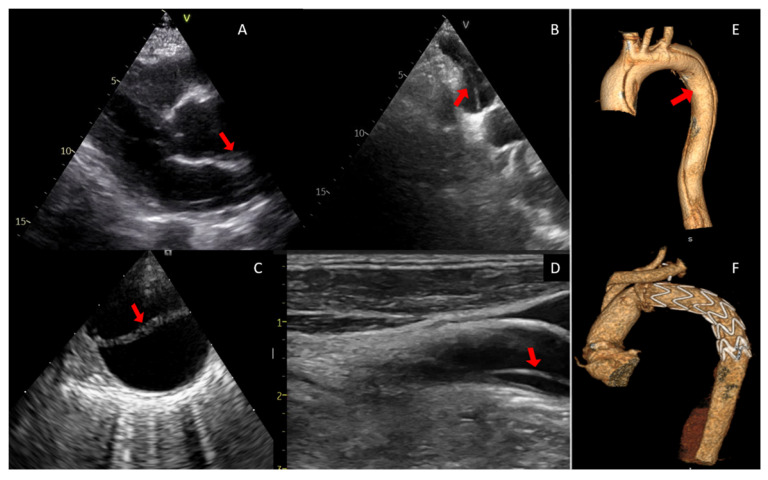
(A) TTE, parasternal long-axis view showing the presence of an intimal flap in the ascending aorta (arrow) and the correct anatomical conformation of the aortic root. (B) Intraoperative suprasternal section of the aortic arch showing an intimal dissection flap and the passage of the guidewire in the aortic arch (arrow) for the correct hybrid (endovascular/surgical) positioning of the prosthesis in the true lumen. (C) Intraoperative TEE, in short axis view of the aorta, revealing a double lumen in the descending aorta and the presence of the intimal flap (arrow). (D) Color Doppler ultrasound of the SAV showing evidence of dissection extending to the carotid artery (arrow). (E) CTA-3D reconstruction of an aortic dissection extending from the aortic root to the abdominal aorta, involving the SAV; the arrow indicates the extension of the true lumen in the small curvature of the aortic arch. (F) 3D CTA reconstruction after surgical repair of the ascending aorta and aortic arch using the FET technique and hybrid prosthesis, necessary to assess the correct functioning and positioning of the prosthesis.
